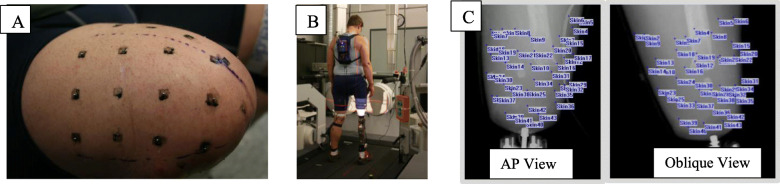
Method for measuring skin strain. A Metal beads were placed in a grid pattern on the skin of the residual femur. B Synchronized biplane radiographs were collected during treadmill walking. C Each bead was identified on every pair of synchronized radiographs to measure skin deformation during gait
